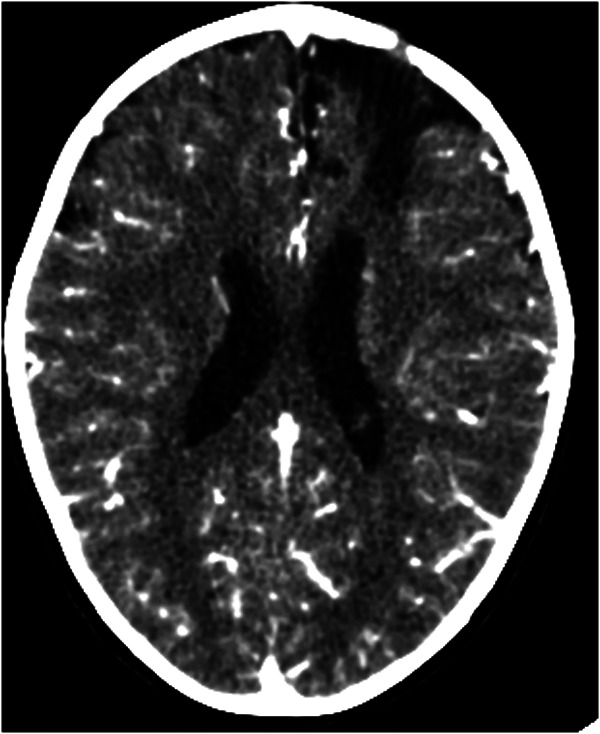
Postoperative contrast-enhanced cranial computed tomography (CT). An axial contrast-enhanced cranial CT scan obtained after surgical drainage of the cerebral abscess shows a reduction of the previously described mass effect, partial resolution of the midline shift, and improved ventricular symmetry. Residual postoperative changes and surrounding edema are observed in the left frontotemporal region.
